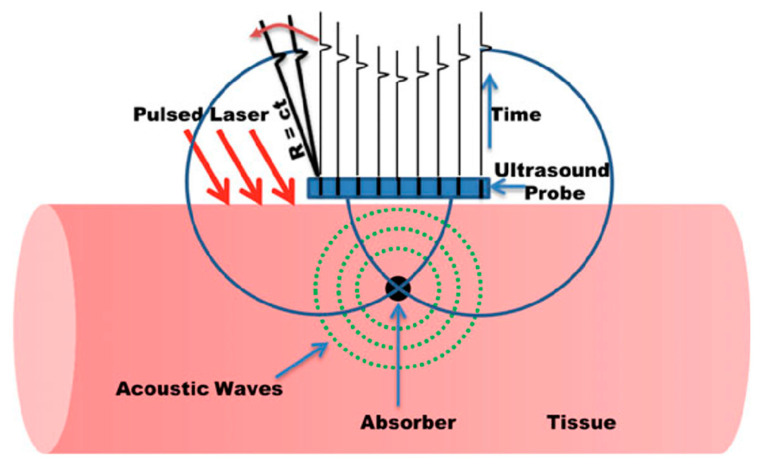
Back-projection PAI reconstruction for a planar detection geometry. Reproduced with permission from Handheld Probe-Based Dual Mode Ultrasound/Photoacoustics for Biomedical Imaging. In: Olivo M., Dinish U. (Eds) Frontiers in Biophotonics for Translational Medicine. Progress in Optical Science and Photonics, volume 3. Springer, Singapore (2016). Copyright 2016 Springer, Singapore.
